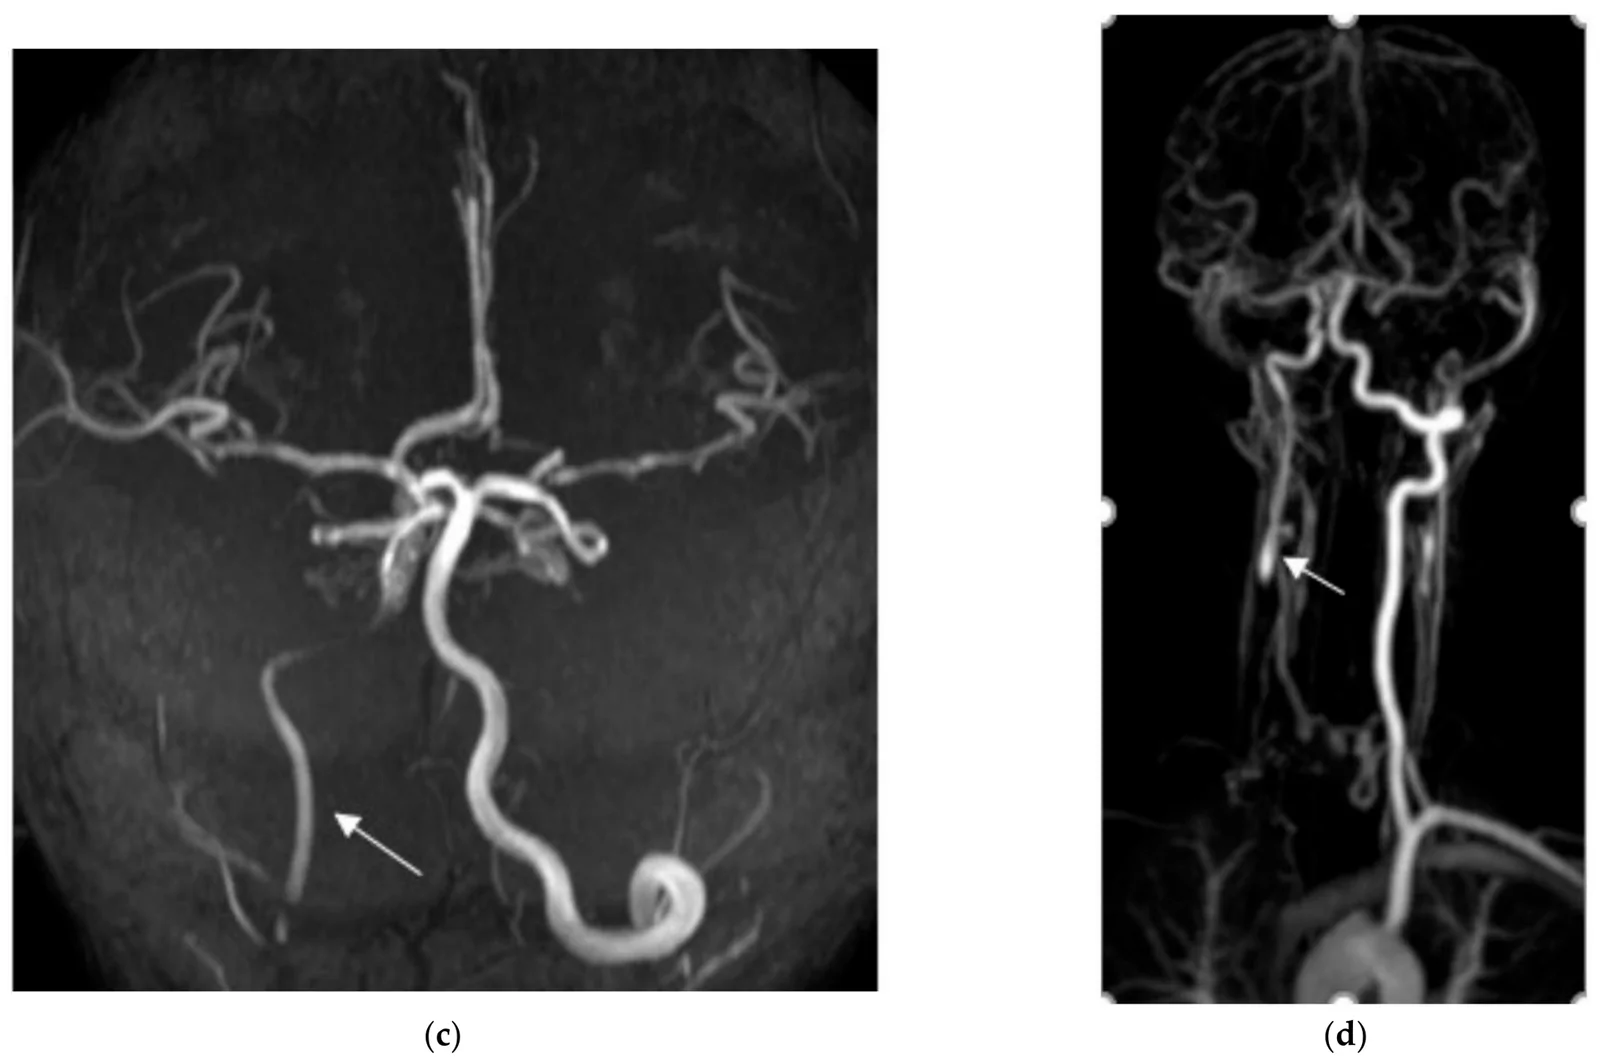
Neuroimaging findings in Case 1: a 13-year-old girl presenting with acute left hemisphere ischemia due to Takayasu arteritis. (a) Diffusion-weighted imaging (DWI) shows increased intensity in the left basal ganglia consistent with acute infarction. (b) Axial FLAIR image shows an area of cytotoxic edema resulting from an acute infarction in the left basal ganglia. (c) Intracranial magnetic resonance angiography (MRA) demonstrates collateral filling of the left middle cerebral artery (MCA) and anterior cerebral artery (ACA) through the circle of Willis. (d) Neck magnetic resonance angiography (MRA) demonstrates occlusion of the left common carotid artery from its origin. The right common carotid artery is filled via collateral circulation from the thyrocervical trunk of the left subclavian artery. The aortic arch gives rise only to the left subclavian artery. The right vertebral artery is partially filled via collateral circulation.
